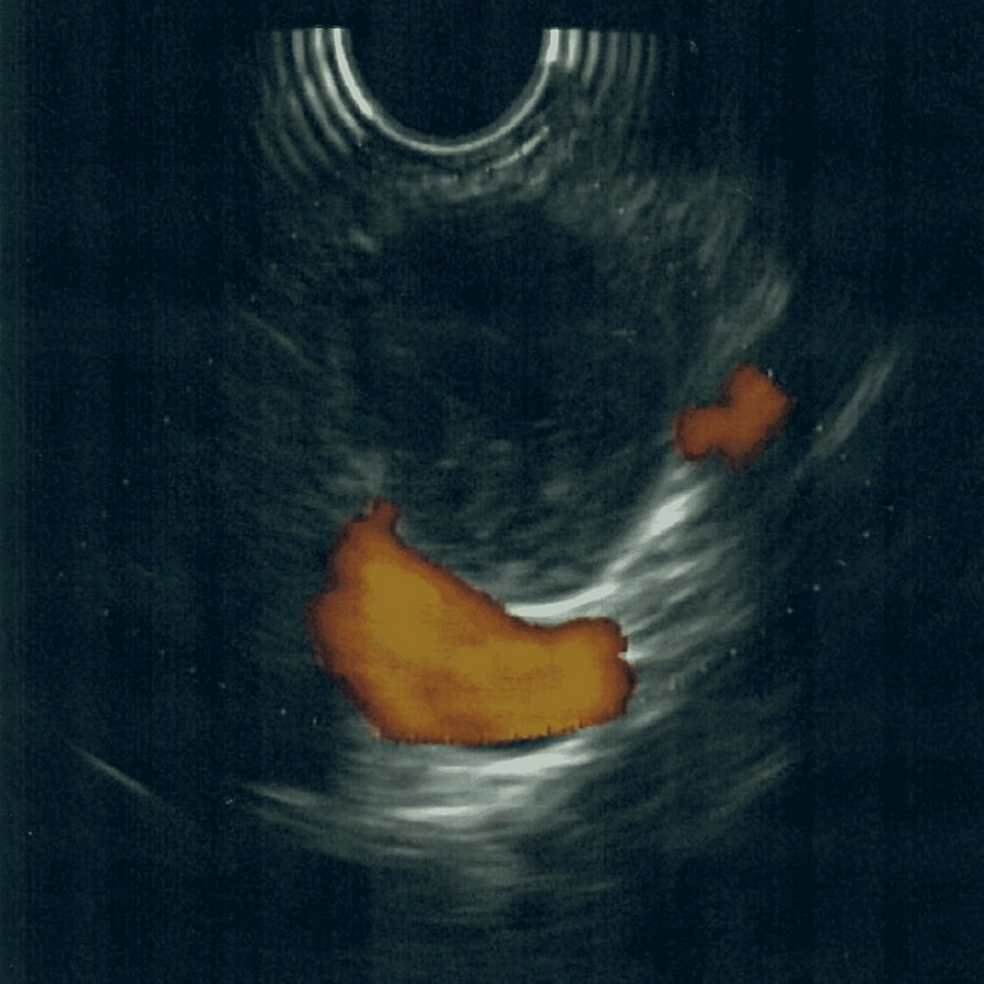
Partially cystic and solid pancreatic schwannoma on endoscopic ultrasound with Doppler.
